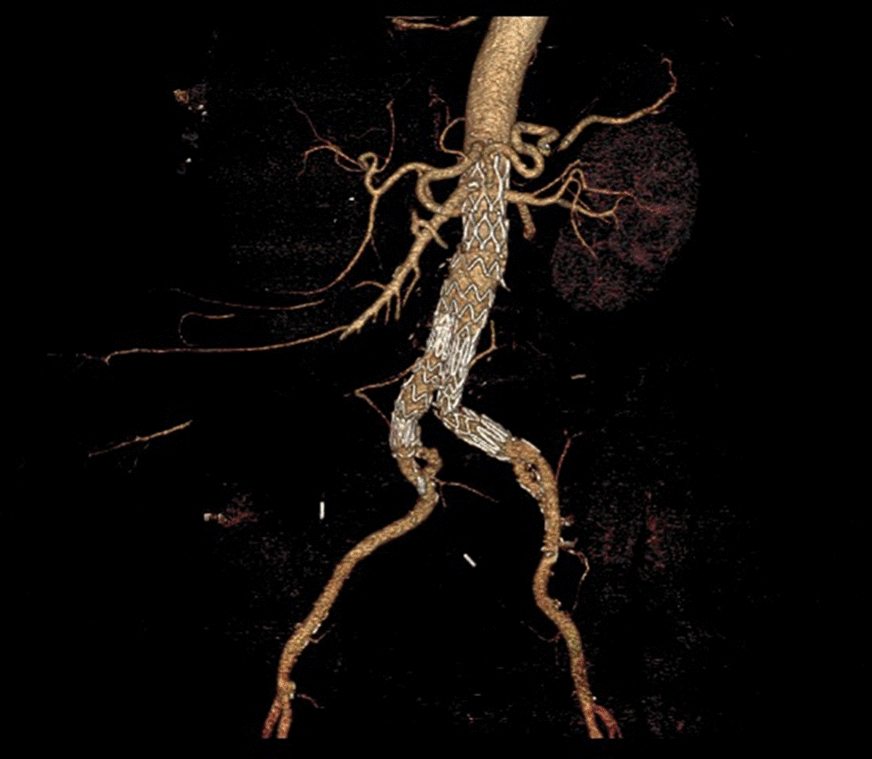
Postoperative CT-scan after initial EVAR
